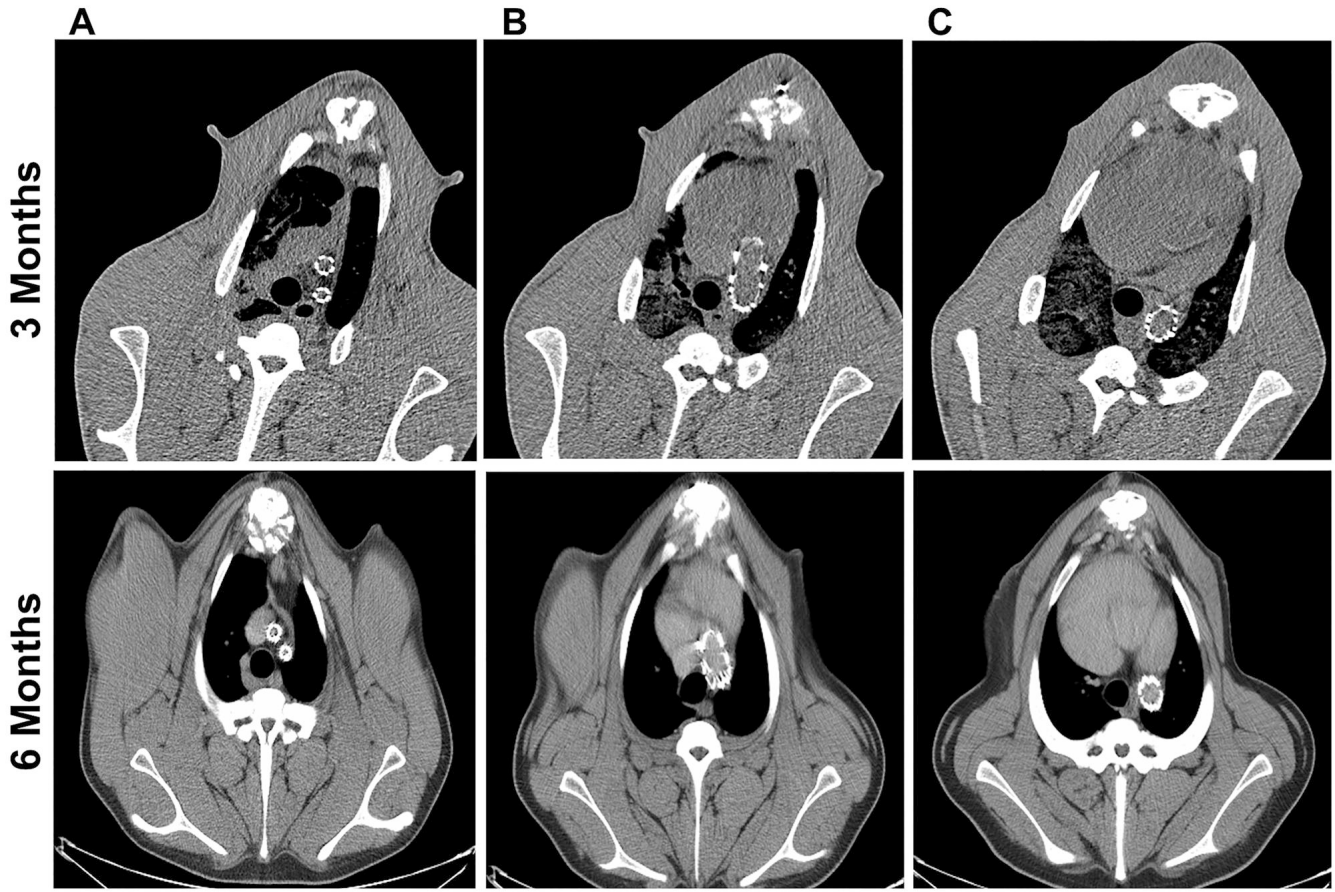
Computed tomography scans taken 90 and 180 days after implanting the double-branched stent graft system revealed no stent fracture or migration. (A) Brachiocephalic branch level; (B) arch level; (C) descending aortic level.
